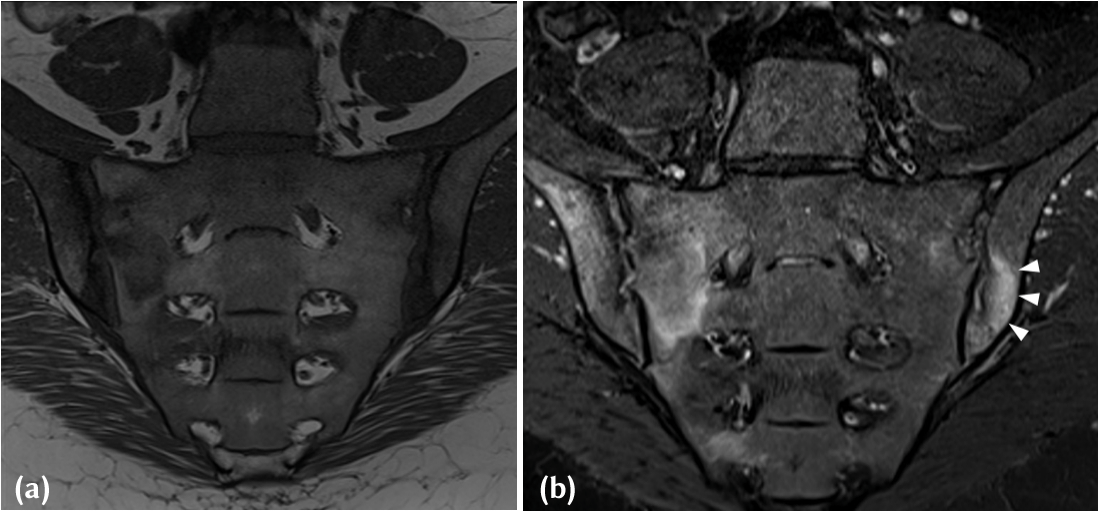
(a) T1-W Mid-coronal MRI in a 38-year-old HLAB27 positive, Female patient who presented with inflammatory back pain and buttock pain. Areas of joint space widening with iliac sided erosions and adjacent marrow low signal intensity are noted in keeping with active inflammatory lesions. (b) Corresponding STIR image in a 38-year-old HLAB27 positive, Female patient who presented with inflammatory back pain and buttock pain, demonstrates extensive high signal intensity (white arrowheads) in the adjacent subchondral region and marrow in keeping with inflammation associated with an active erosion.
